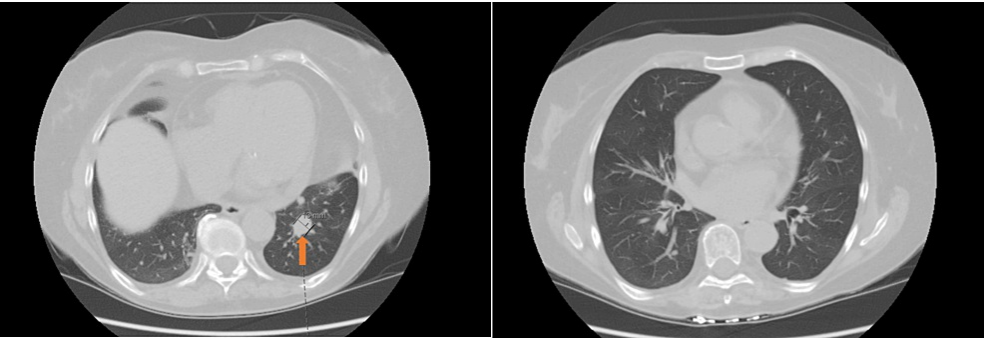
CT chest showing new 13 mm oblong mass in left lingula (left) and tree-in-bud pattern (right)
